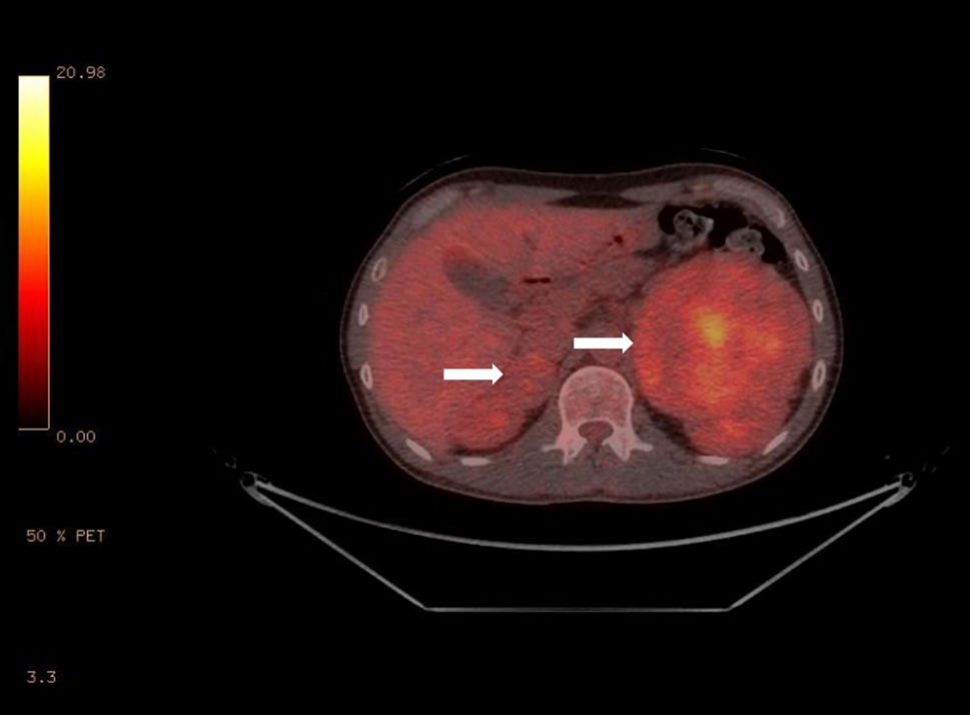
FDG- PET scan showing bilateral adrenal masses with tracer uptake
